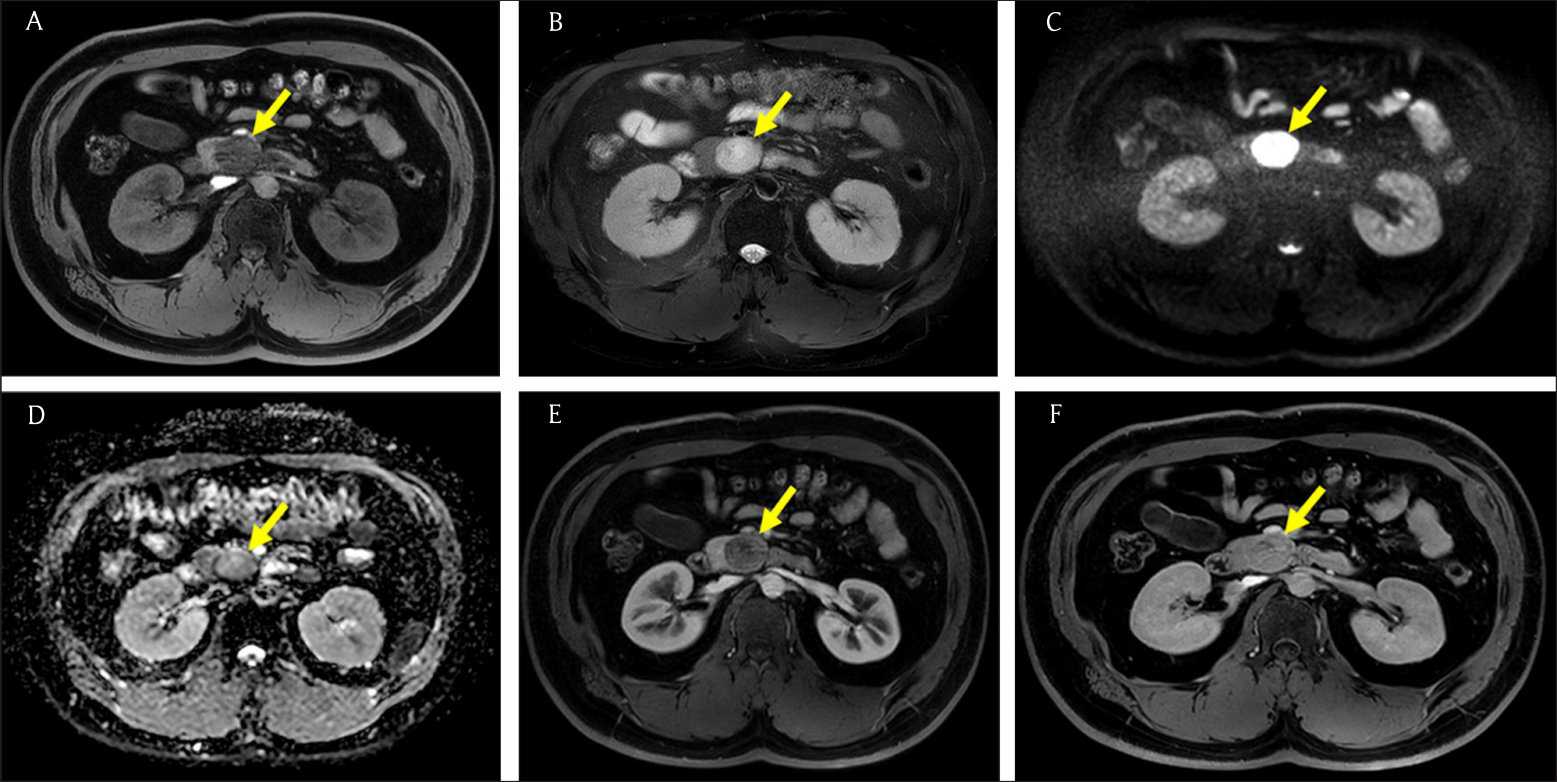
A 31-year-old man with a small solid pseudopapillary tumor. (A) Axial T1-weighted fat-suppressed MR image shows a 3-cm solid mass (arrow) with a well-defined margin in the pancreatic head. (B) On axial T2-weighted fat-suppressed MR image, the mass (arrow) shows relatively homogeneous high signal intensity. (C, D) Axial diffusion-weighted image (b = 800 s/mm^2^) and apparent diffusion coefficient map show the mass (arrow) with diffusion restriction. (E, F) Axial gadolinium-enhanced arterial and 3-minute delayed phase MR images show early heterogeneous and progressive enhancement of the mass (arrow).
